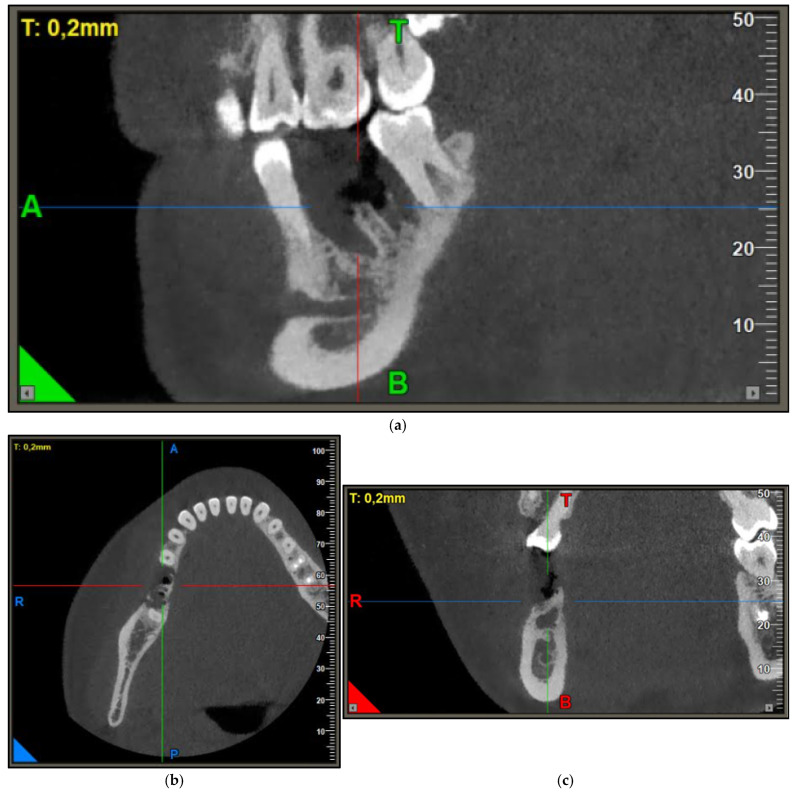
Pre-operative CBCT of necrotic site: (a) Extraction socket of tooth 46, devitalized bone region 45–47, sagittal view of the situation. (b) Axial view. (c) Coronal view.
